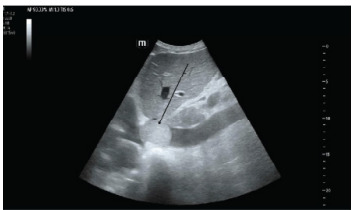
Point-of-care ultrasound of the inferior vena cava (IVC) showing a hyperechoic circular mass in the proximal IVC (arrow) adjacent to the right atrium. Distal IVC is seen plethoric.
